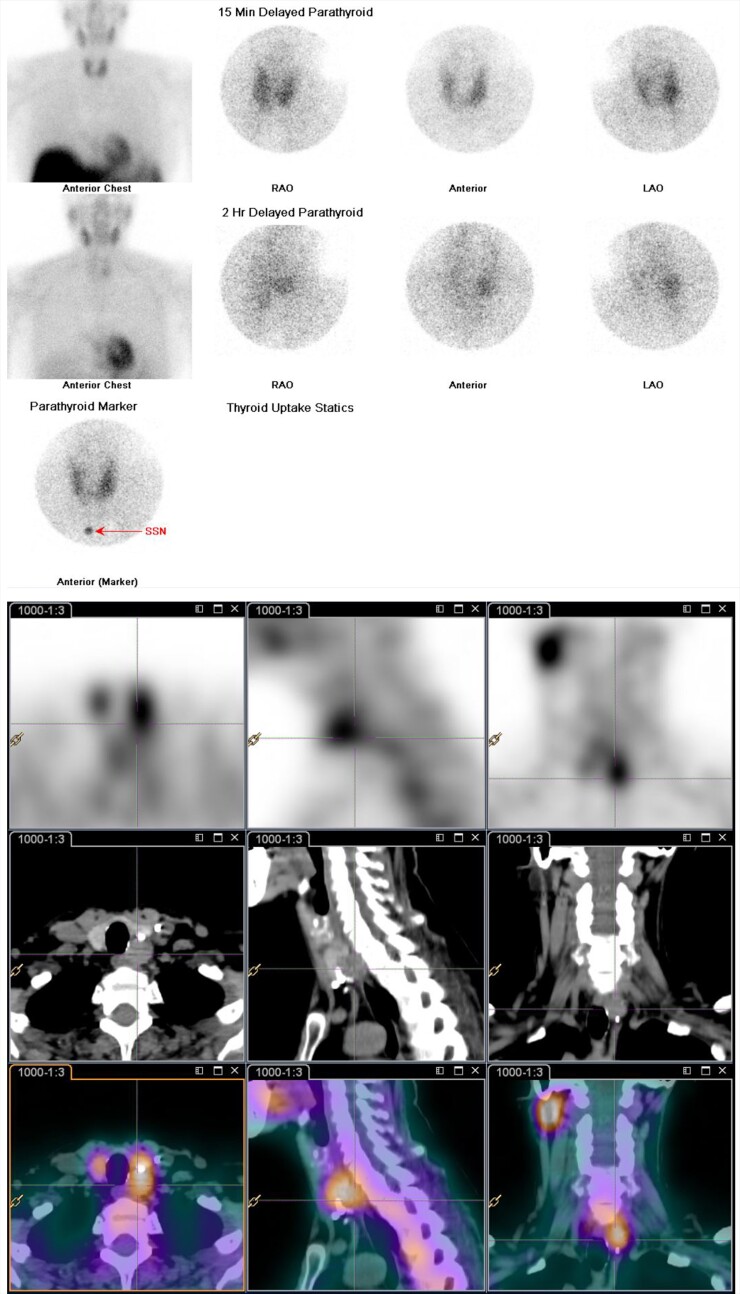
Parathyroid scintigraphy was performed including planar, pinhole, and SPECT/CT imaging following intravenous injection of radiolabeled MIBI in 2021 at first presentation of dysphonia and hyperparathyroidism. There was focal increased MIBI uptake in a 17-mm solid rounded nodule lying posterior to the lower pole of the left lobe of the thyroid. There were a number of focal high attenuating areas lying anterior and inferior to the nodule in keeping with surgical clips. In the 2-hour delayed imaging, there was washout of activity from the thyroid and preferential retention of tracer at the side of the nodule in the left neck. This was in keeping with a left inferior parathyroid nodule. There were no other sites of uptake seen.
